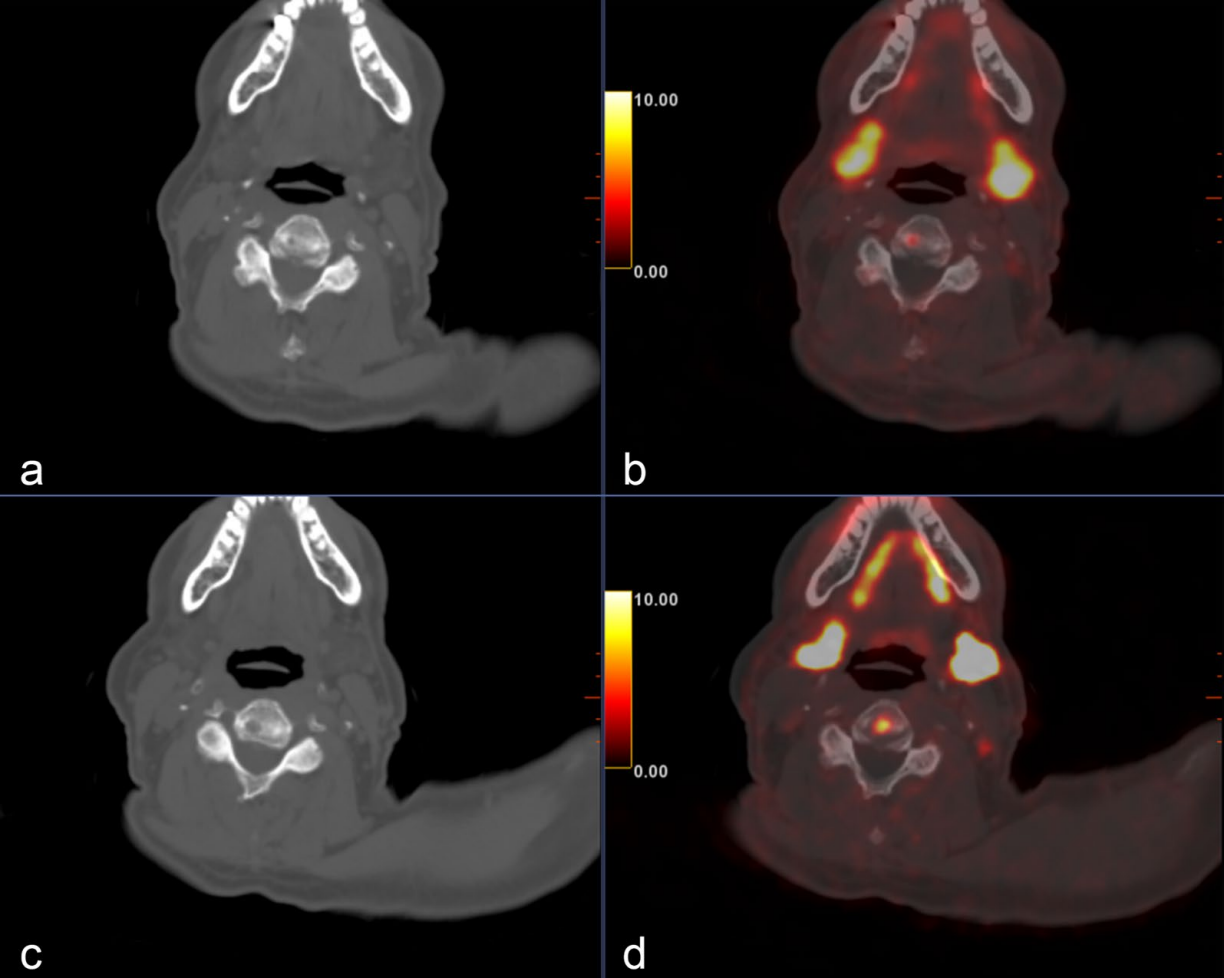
A 69-year-old patient (patient number 9) with unfavorable intermediate risk PC, who had PSA persistence after undergoing an RP. a Staging PET/CT, axial CT, and fused image showing a small lucent lesion in a C4 vertebral body with mild focal PSMA uptake, classified as PSMA-RADS 3B. b Postoperative PET/CT, axial CT, and fused image showing enlargement of the same lesion with more intense uptake. This patient had also a pelvic PSMA-RADS 3B lesion that remained unchanged. An MRI of the pelvis and spine supported these findings (not shown)
